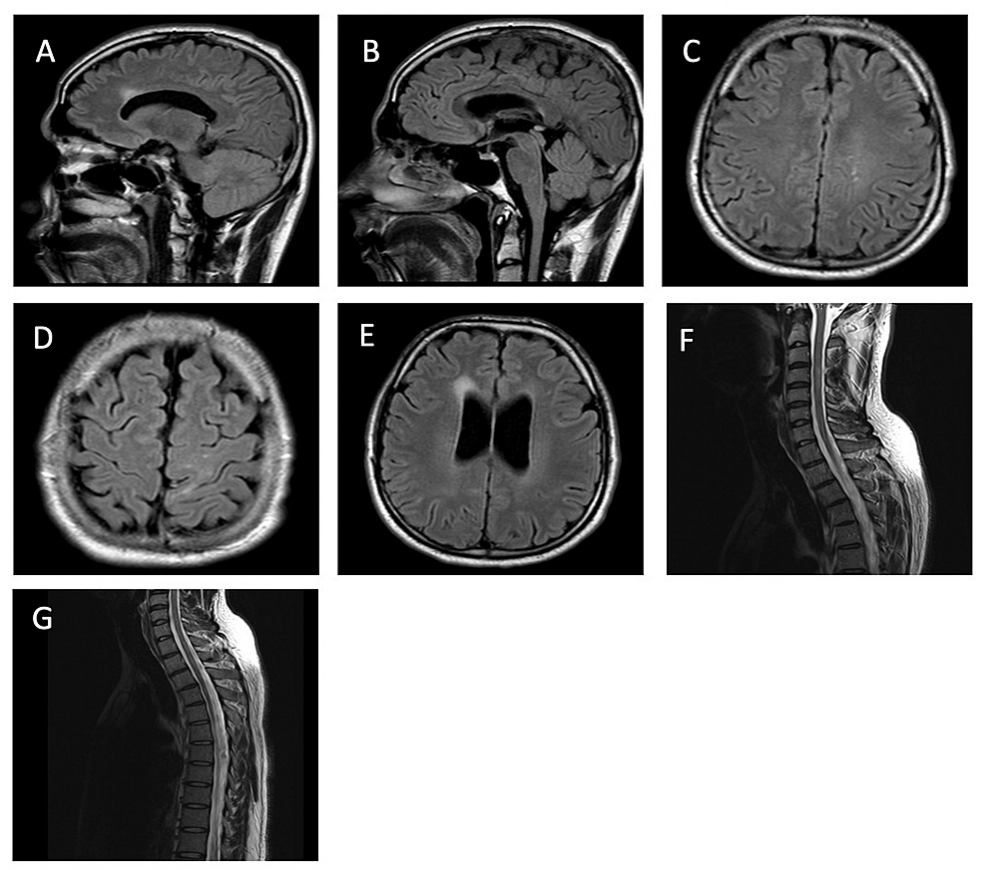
MRI brain and spinal cord. (A-B): Sagittal view of brain MRI (FLAIR sequence) showing multiple hyperintense signal changes in the corpus callosum. (C-E): Axial view of the brain MRI (FLAIR sequence) showing left cortical (parietal), left subcortical, and deep periventricular hyperintense signal changes, respectively. (F-G): Sagittal view of spine MRI showing multilevel eccentric signal changes of the spinal cord. MRI: magnetic resonance imaging; FLAIR: fluid-attenuated inversion recovery
